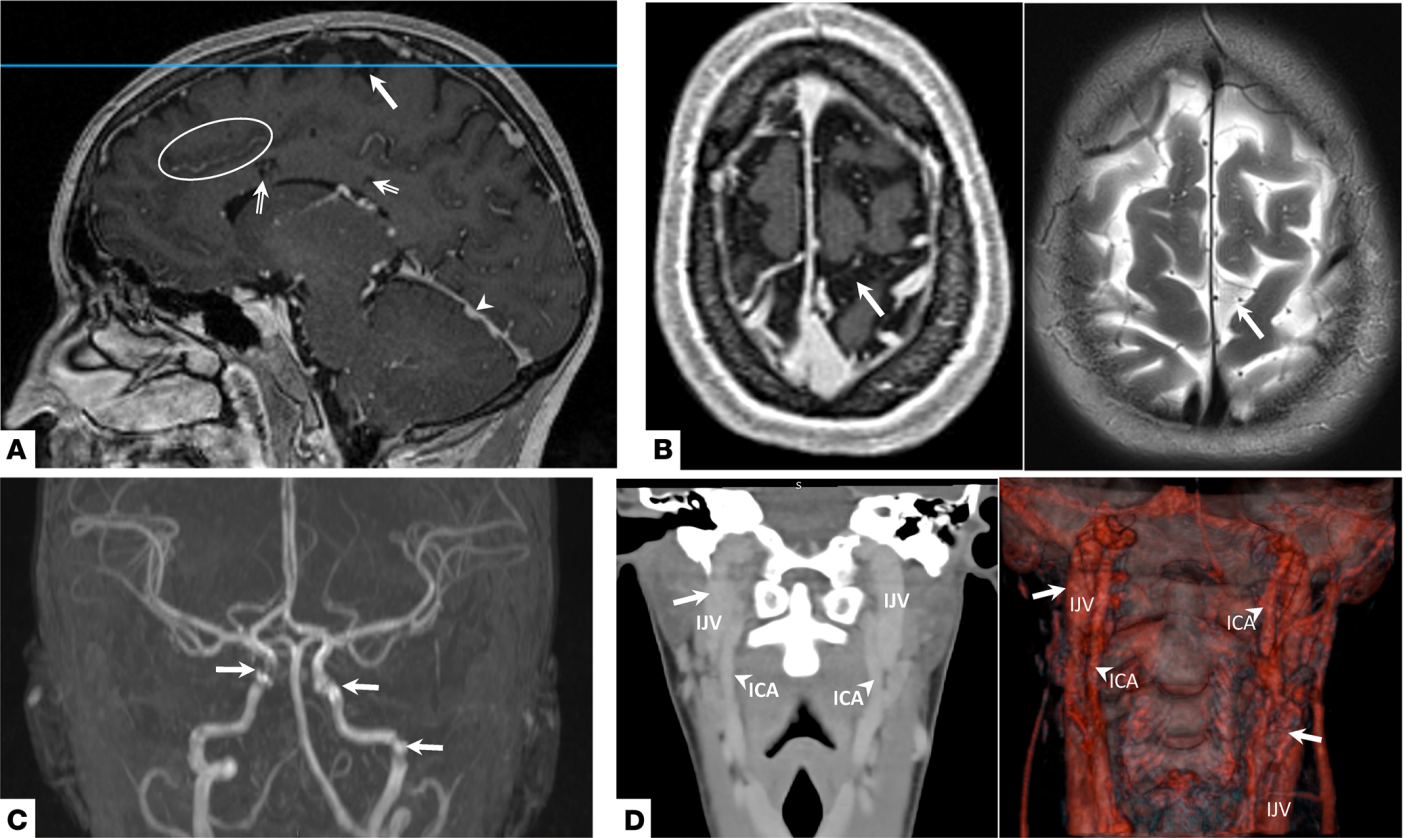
Intracranial malformations and rete mirabile in HIF-2α gain-of-function syndrome patients. Representative images are shown. (A) Sagittal T1-weighted postcontrast MRI of the head of patient 1 shows a subarachnoid cavernous angioma (arrow). Prominent interstitial fluid or Virchow-Robins spaces are seen within the corpus callosum (double-lined arrows); these are seen throughout the parenchyma following veins (white circle). Prominent tentorial veins are also seen (arrowhead). (B) Axial T1-FLAIR (left) and T2-weighted (right) sequences of the same patient show the subarachnoid cavernous angioma (arrow) arising from veins. This corresponds to the blue line in A. (C) Reconstruction of TOF MR angiogram of patient 3 shows dysplastic segments of the internal carotid artery bilaterally (arrows). (D) Coronal CT of the neck with contrast of the same patient shows a plexiform jugular vein surrounding a dysplastic carotid, known as a rete mirabile, at the cranio-cervical junction on the right (arrow); volumetric reconstruction of the same CT of the neck demonstrating rete mirabile on the right and left (arrows). ICA, internal carotid artery; IJV, internal jugular vein.
